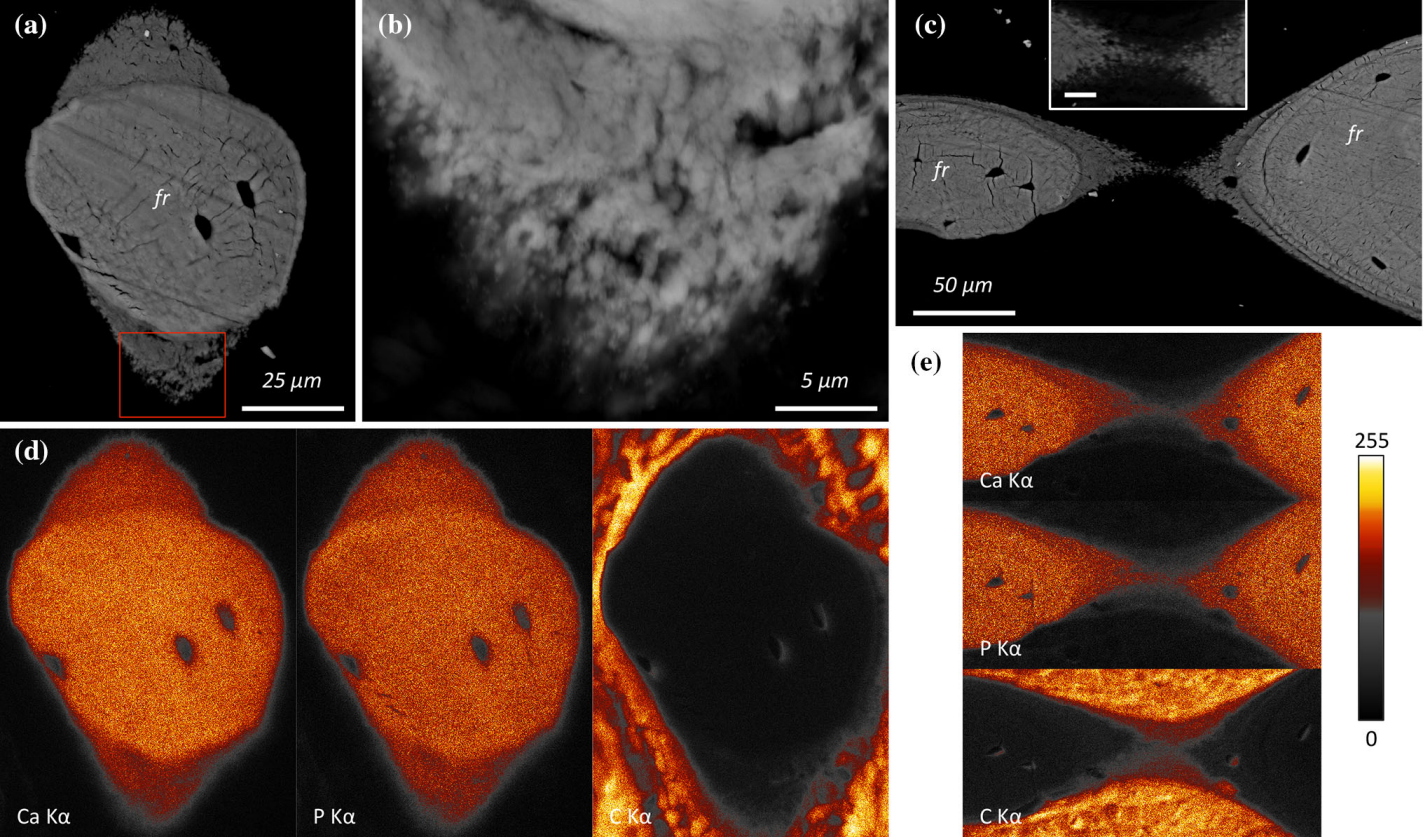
a, b The mineralisation front appears granular. c The newly formed bone bridges the gap between two fragments. *Inset:* Detail of the granular mineralisation front (*Scale bar* 10 µm). d, e Elemental maps representing the Kα X-ray emission lines for calcium (Ca Kα), phosphorus (P Kα), and carbon (C Kα) corresponding to (a) and (c), respectively, reveal lower Ca and P content of the newly formed bone compared to the old bone of the fragment
